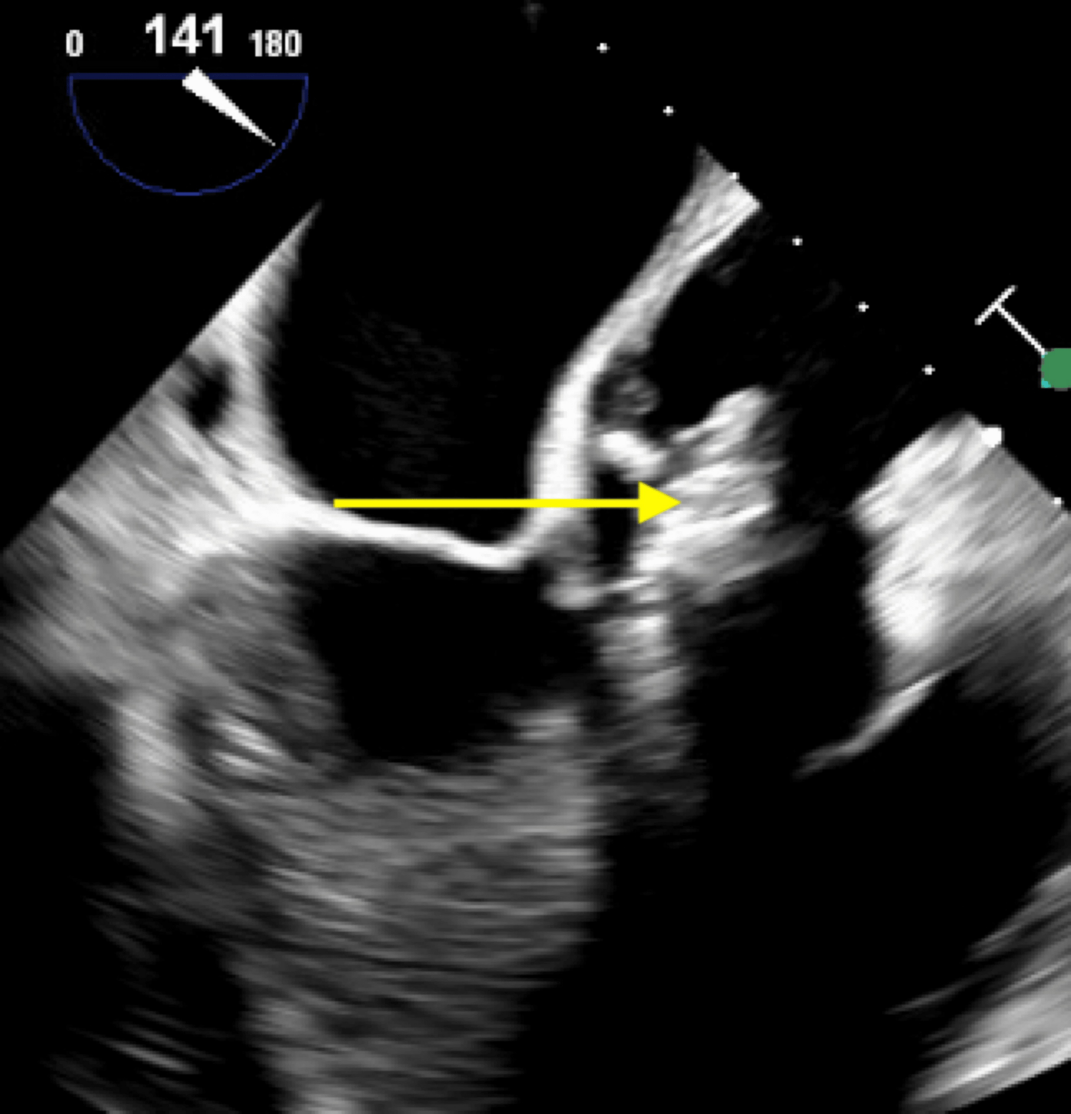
Transesophageal echocardiogram demonstrating a bulky, echodense lesion measuring 2.2 × 1.9 cm adherent to the right cusp (yellow arrow) (mid-esophageal long-axis (ME LAX) view).
